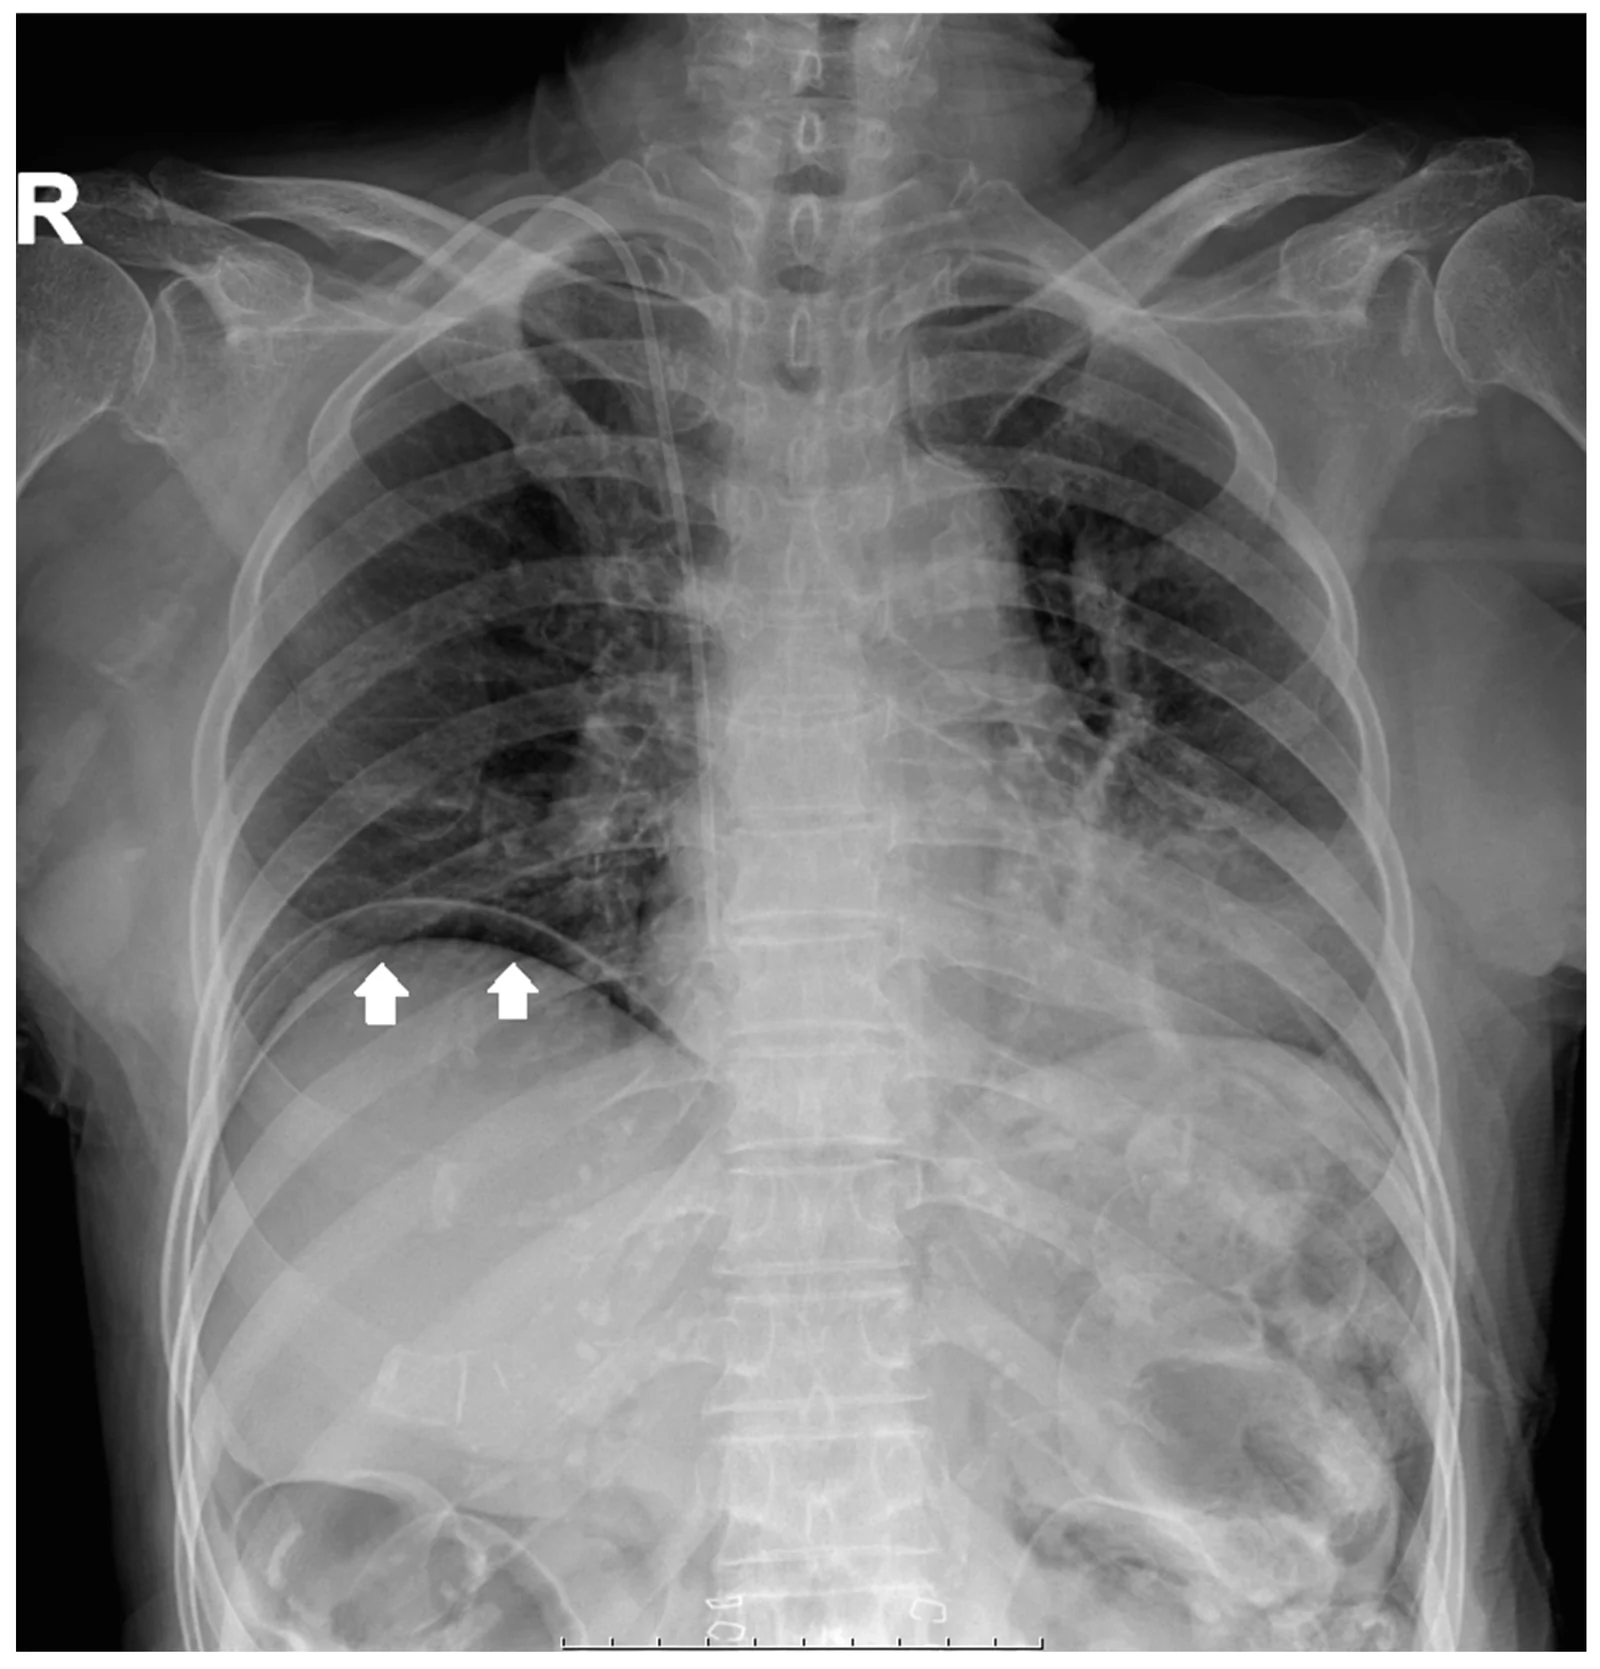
Portable anteroposterior chest radiograph obtained on postoperative day 2, demonstrating free air beneath the left hemidiaphragm (arrows), indicative of pneumoperitoneum. ‘R’ indicates the patient’s right side.
